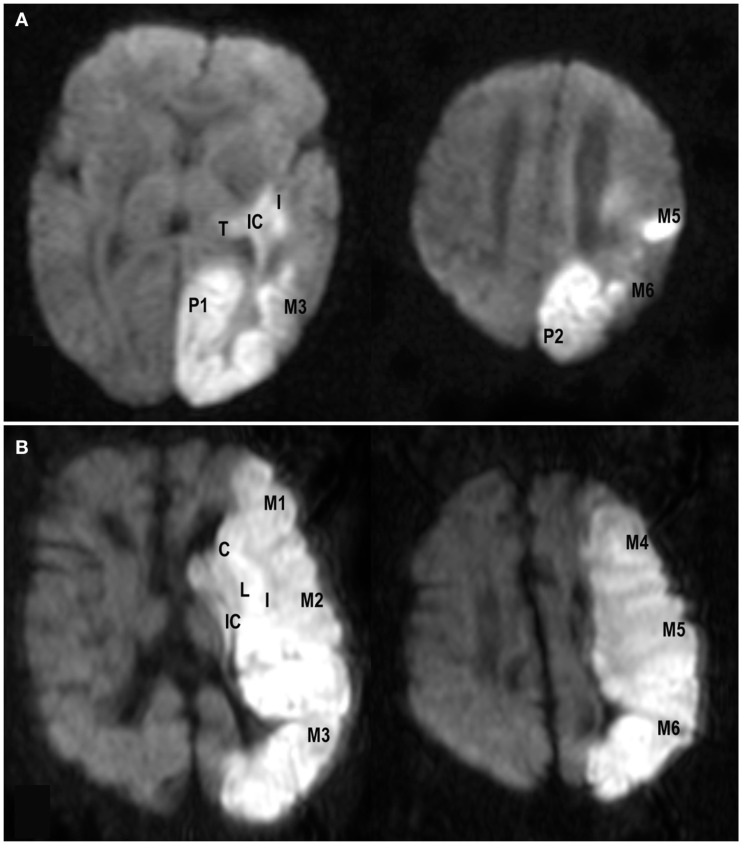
Examples of axial diffusion weighted images from a neonate (A) with a modified pediatric ASPECTS of eight (involvement of left internal capsule, insula, M3, M5, M6, P1, P2, and thalamus) and a child (B) with a modified pediatric ASPECTS of 10 (involvement of left caudate, lentiform, internal capsule, insula, and middle cerebral artery territories M1–M6).
